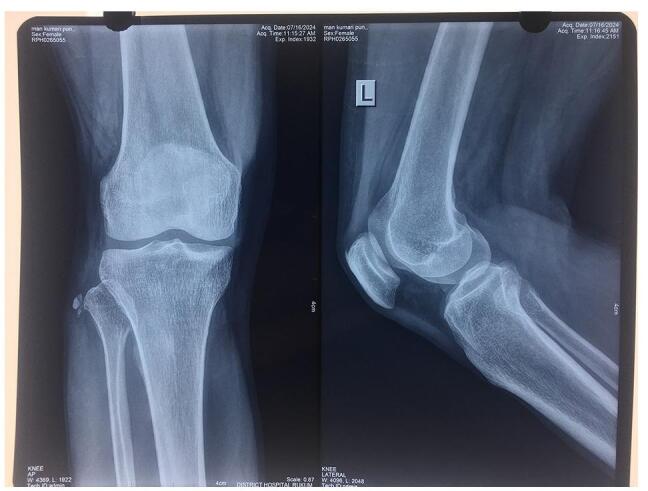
Antero-posterior and lateral knee X-rays demonstrate multifocal calcium deposits at the lateral aspect of the proximal fibula.
